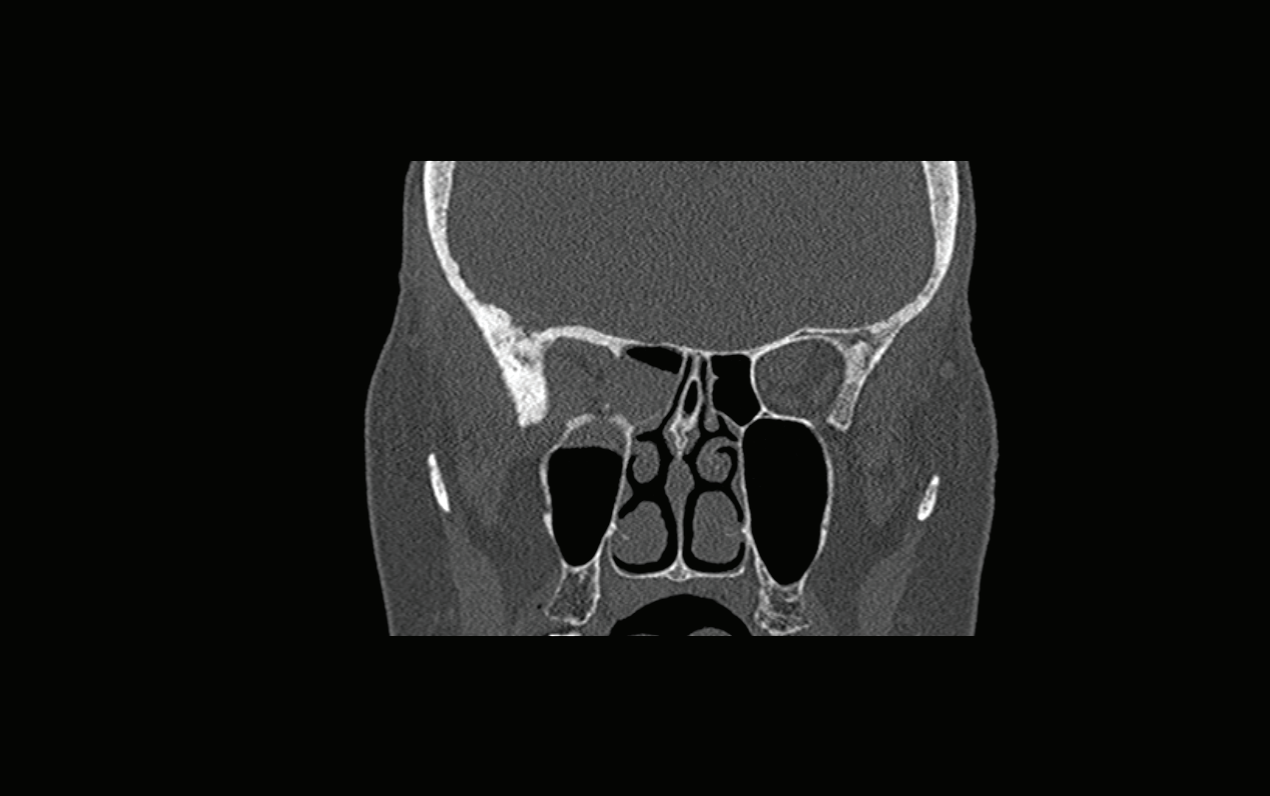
Coronal CT scan showing non-specific findings of “mucosal disease” involving the right maxillary, ethmoid, and sphenoid sinuses. An erosion of the lateral wall of right sphenoid is also noted.
